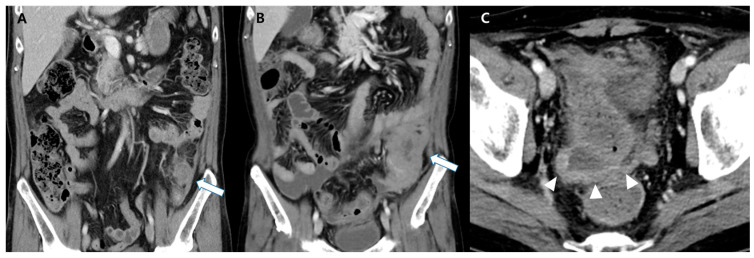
(A) Initial contrast-enhanced computed tomography image. This image shows rim-enhancing fluid collection with adjacent inflammatory changes localized in the juxta-descending colon, mimicking a paracolic abscess (arrow). (B) Follow-up scans obtained five weeks later. This follow-up image demonstrates the rapid and unexpected development of a large soft-tissue mass (arrow) in the descending colon (previously the site of the suspected abscess). Additionally, a small amount of ascites with nodular peritoneal thickening was observed (arrowheads). This finding prompted further investigations. (C) Additionally, a small amount of ascites with nodular peritoneal thickening was observed (arrowheads). This finding prompted further investigations.
